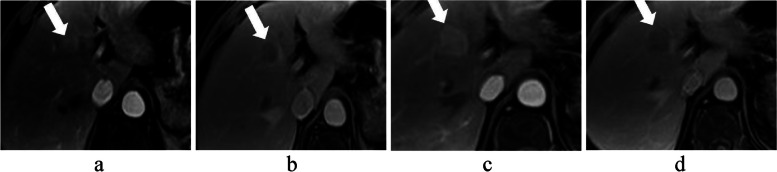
A 54-year-old man with HCC (biopsy pathology). EOB-MRI performed on July 25, 2017 demonstrated an 18-mm observation (arrow) in S4 of the liver with nonrim APHE (a) and washout on PVP (b). Then, EOB-MRI performed on September 5, 2017 (less than 2 months after the prior exam) showed that the nodule had grown to 29 mm with nonrim APHE (c), washout and an enhancing capsule on the PVP (d)
